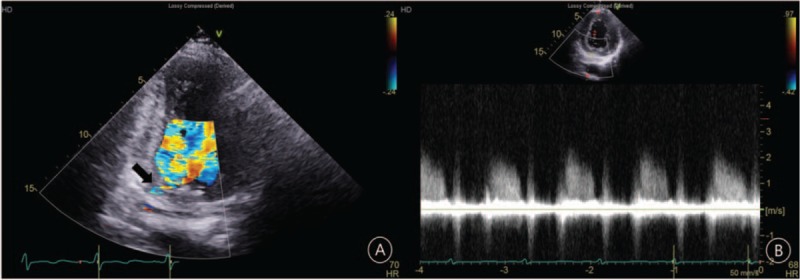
The modified apical 2-chamber view showing the shunt flow (black arrow) into the left ventricle (A). Continuous-wave Doppler recording of the shunt flow (B).
